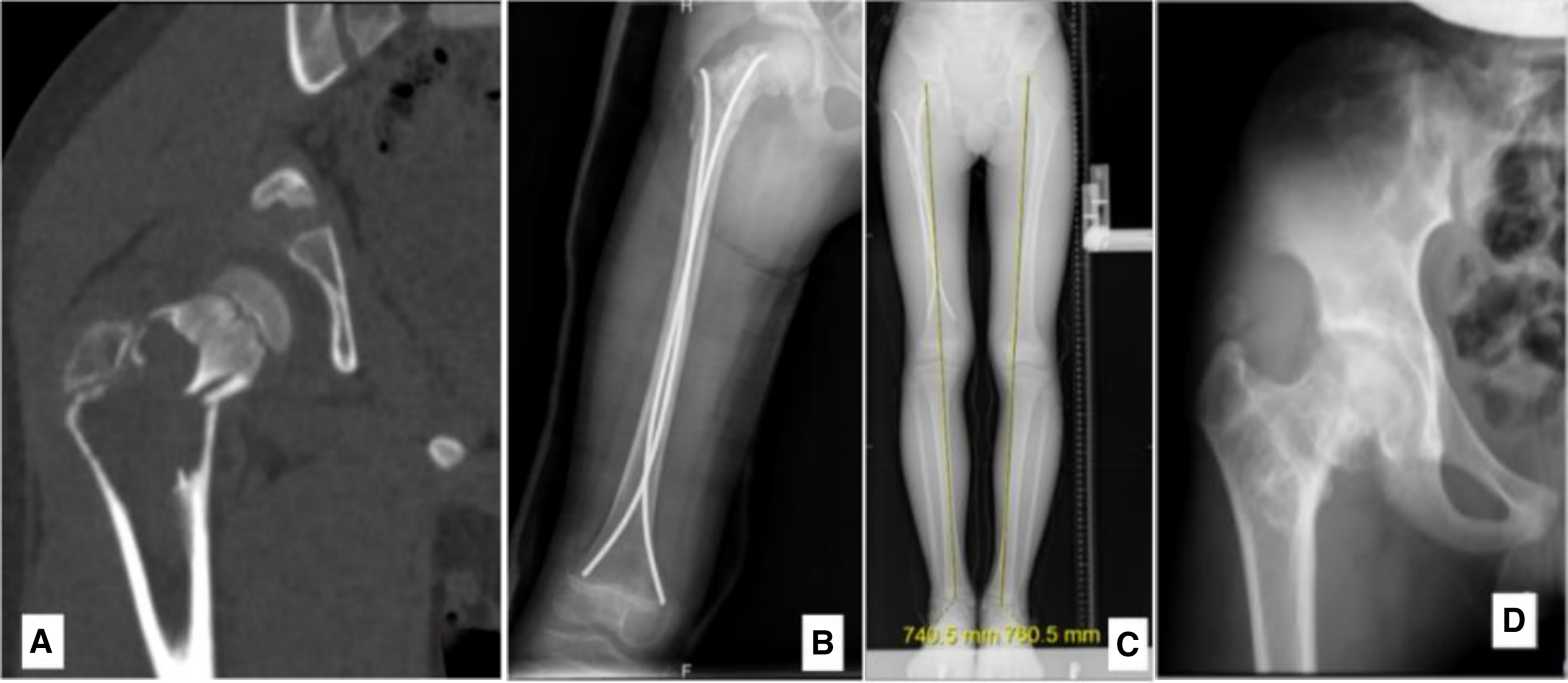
An 8.7-year-old male diagnosed with an ABC in the right proximal femur, accompanied by a fracture. (A) Preoperative CT scan, coronal section. (B) Radiograph taken 3 days post-biopsy, curettage, FIN procedure, and bone grafting. (C) Radiograph at 2.2 years post-surgery showing LLD with the right femur being 2 cm shorter. (D) Radiograph at 5.4 years post-surgery, highlighting premature closure of the epiphysis, deformity due to femoral neck shortening, elevated position of the greater trochanter (high riding), and coxa vara.
